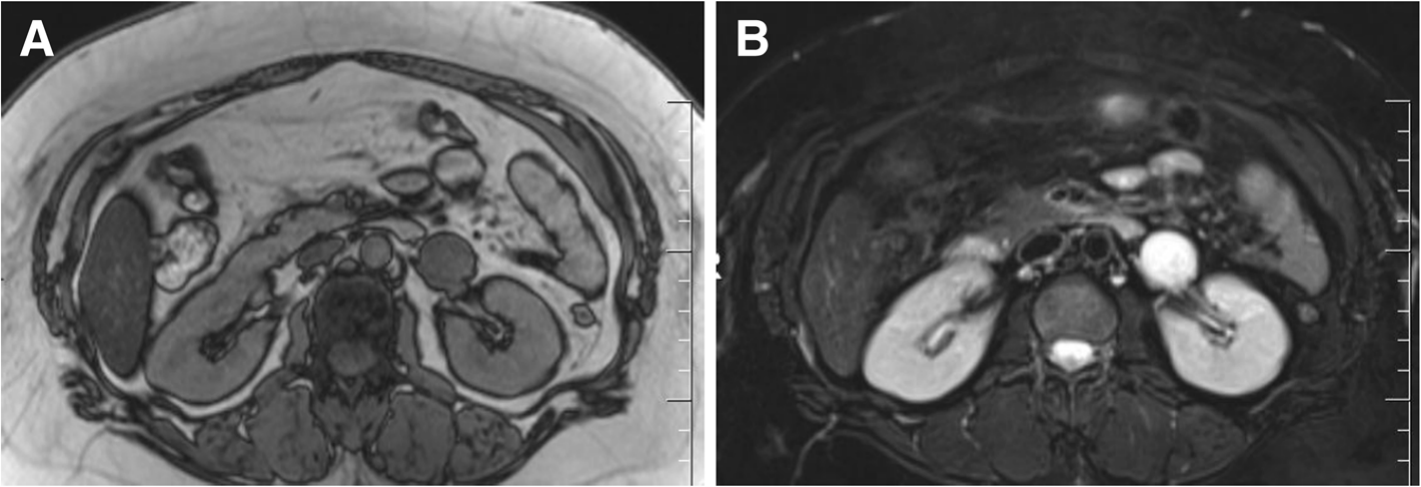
Magnetic resonance imaging findings. (A) T1-weighted images showed a tumor with low signal intensity and (B) very high signal intensity on T2-weighted images.
